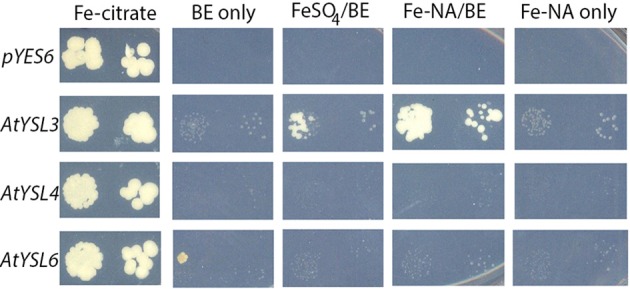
Neither *AtYSL4* nor *AtYSL6* is able to complement *fet3fet4*. These experiments were performed as described (Chu et al. 2010). *fet3fet4* yeast (derived from DEY1453) transformed with *pGEV* and *pYES6/CT* empty vector (top row) or constructs containing *AtYSL3* (middle row), *AtYSL4* (third row), and AtYSL6 (bottom row) were plated onto SD-TRP medium containing 50 mM Fe-Citrate (first column), 0 mM iron + 10 nM beta-estradiol (BE) (second column), 5 mM FeSO4 + 10 nM BE (third column), 5 mM Fe-NA complex + 10 nM BE (fourth column), or 5 mM Fe-NA complex without BE (last column). Pairs of spots correspond to 100 and 1000 fold dilutions of the original cultures. Plates were photographed after 10 days of growth.
